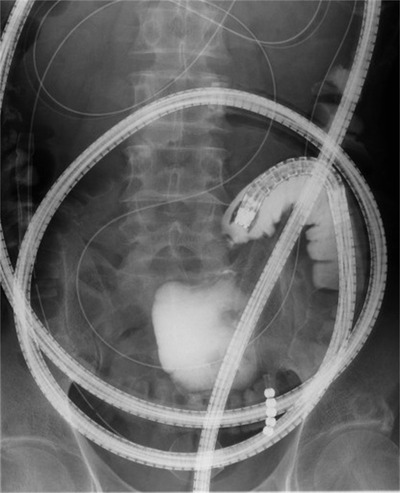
Roentgenography showing obstruction by a tumor. The process was performed using enteroscopy under fluoroscopic guidance
